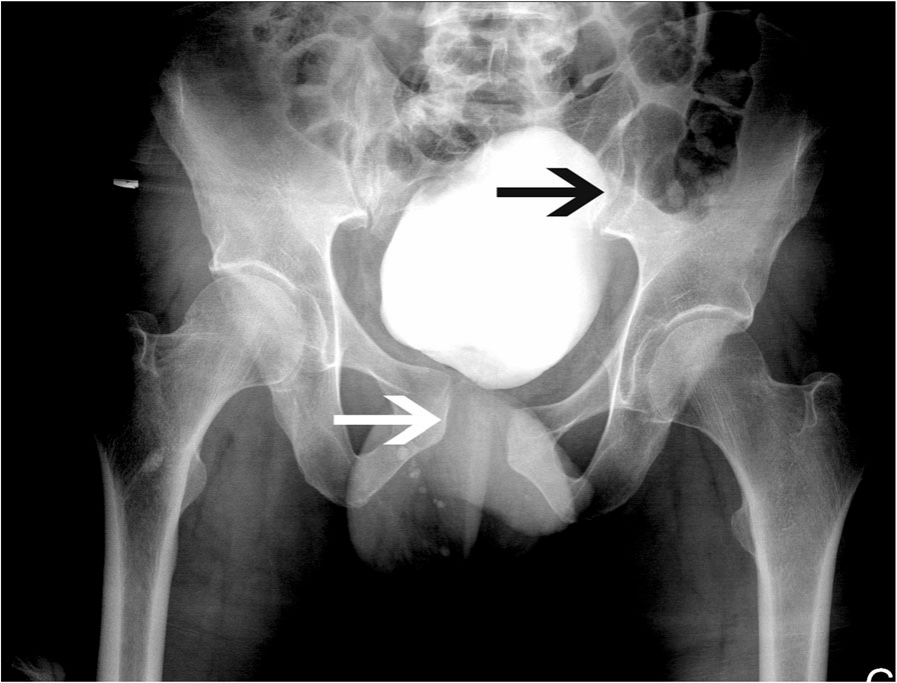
Pelvic anteroposterior plain film after binder removal showing pubic symphysis diastasis (*white arrow*) and left sacroiliac disruption (*black arrow*)
